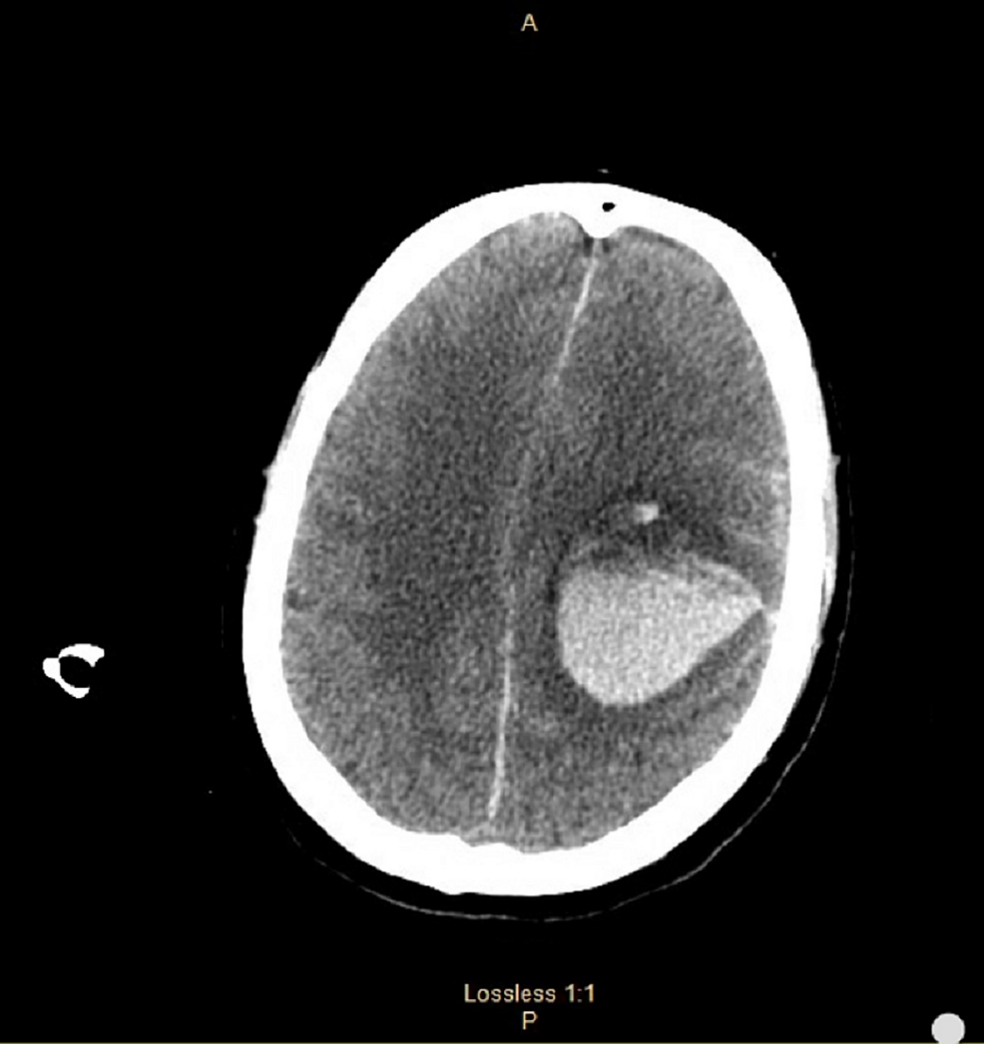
CT of the head without contrast showing extensive intraperitoneal hemorrhage within the left frontoparietal region with extension into the cerebral ventricles.
